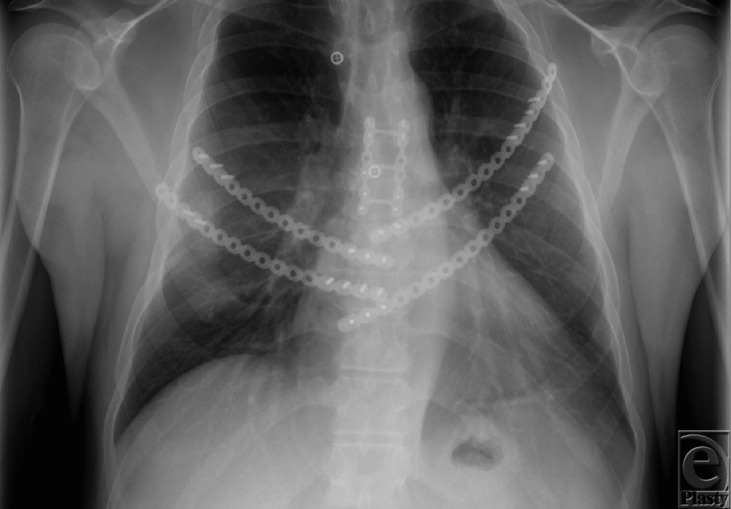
Postoperative posteroanterior chest roentgenogram. Notice the artificial rib technology at the lateral edge of the ribs and midline at the sternum. Also, the ladder plate providing sternal stability for the transverse sternotomy.
